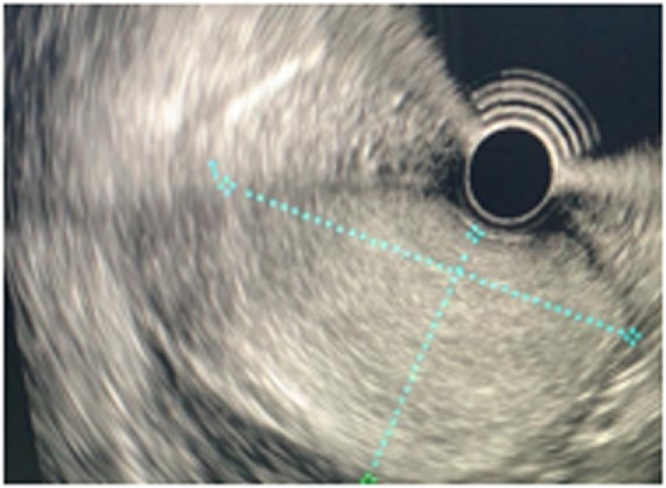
Endoscopic ultrasound: subepithelial lesion with exophytic area.
